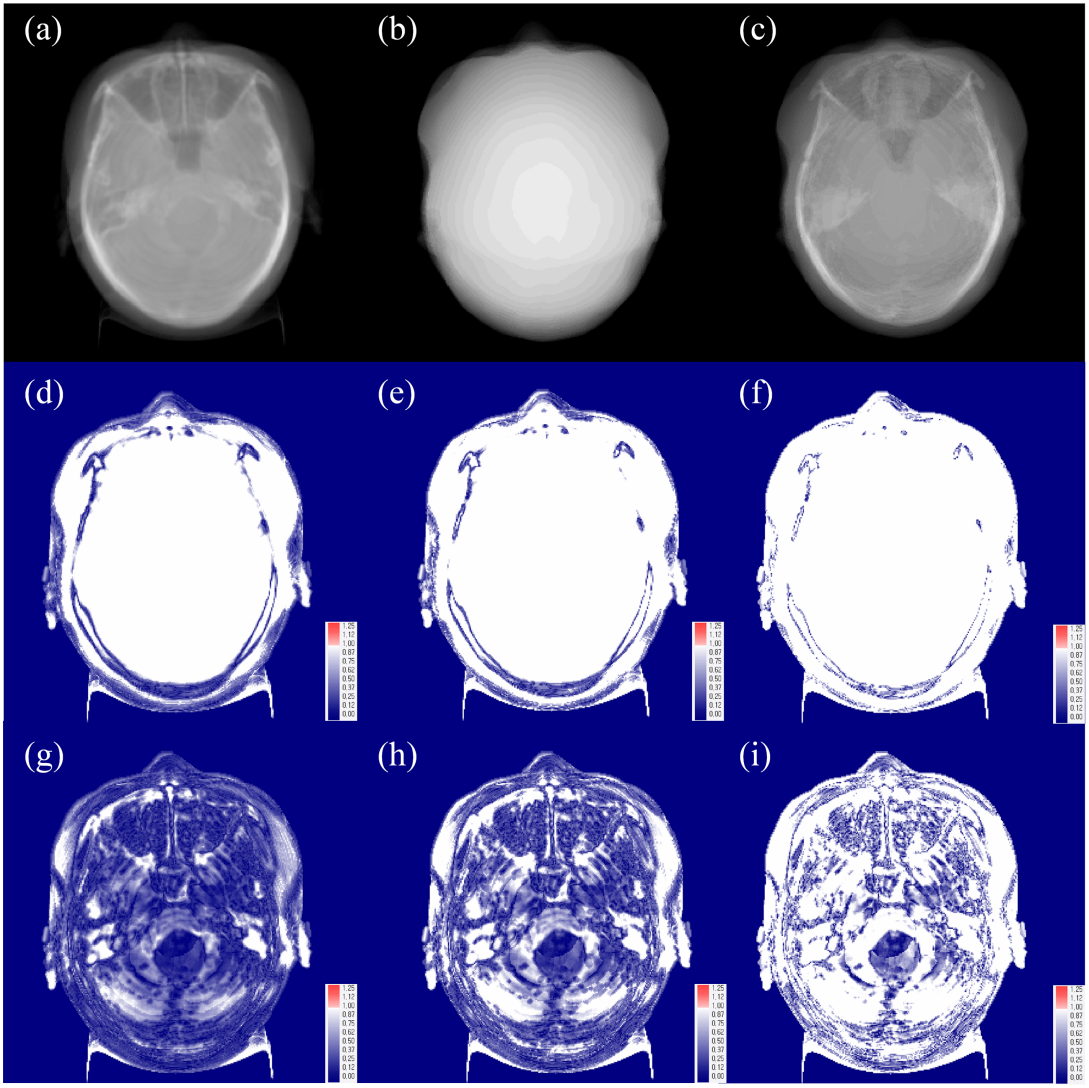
Gamma analysis between the original CT image and resultant synthetic CT images. (a) Original CT image. (b) Water-equivalent synthetic CT (_W_CT). (c) Bulk-density-assigned CT image (_B_CT). (d) Gamma analysis between the original CT and _W_CT with 3 mm/3% criteria. (e) Gamma analysis between the original CT and _W_CT with 2 mm/2% criteria. (f) Gamma analysis between the original CT and _W_CT with 1 mm/1% criteria. (g) Gamma analysis between the original CT and _B_CT with 3 mm/3% criteria. (h) Gamma analysis between the original CT and _B_CT with 2 mm/2% criteria. (i) Gamma analysis between the original CT and _B_CT with 1 mm/1% criteria.
